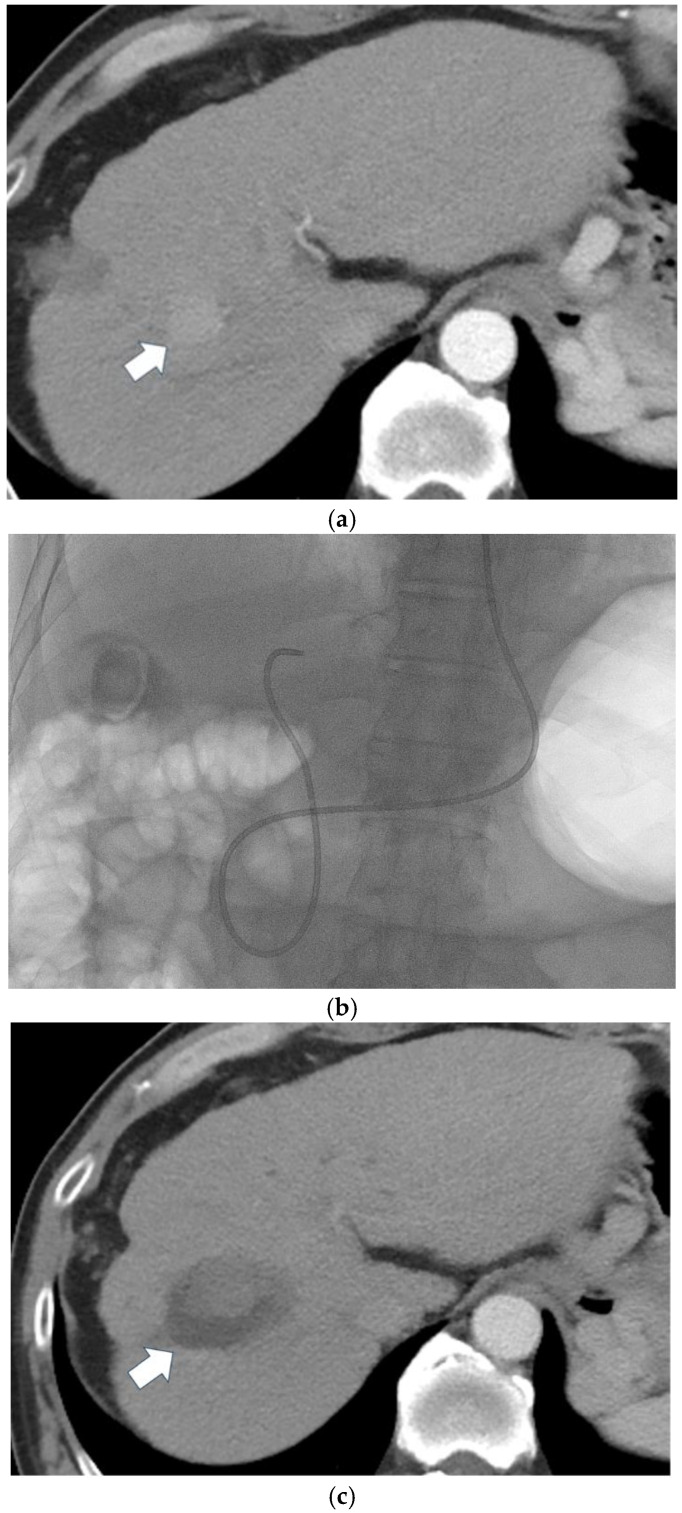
(a) Pre-ablation CT of a 67-year-old man showing a 22 mm central HCC in segment 8 (arrow); (b) ENBD insertion before ablation; (c) post-ablation CT demonstrating complete ablation (arrow).
